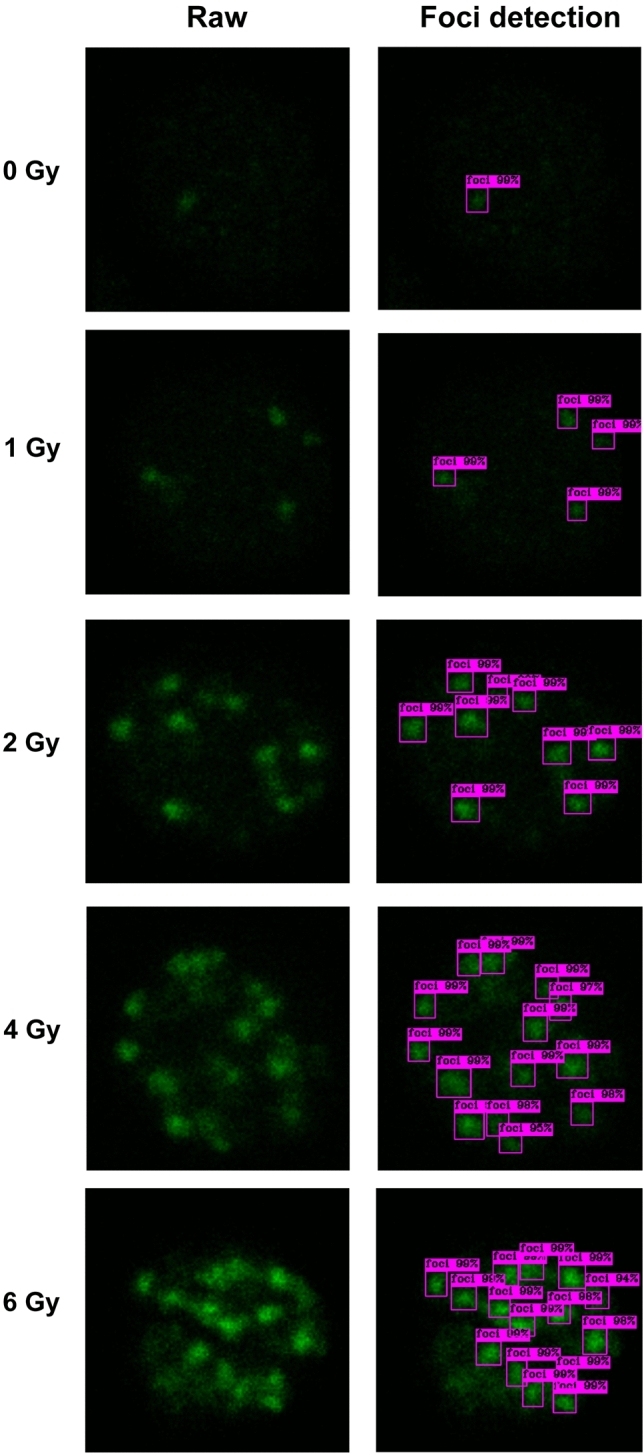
Performance testing of the FociRad. Green channel cropped images of cells irradiated with X-rays (0, 1, 2, 4 or 6 Gy) (left column) and automated foci detection with confidence thresholds by the FociRad model (right column) are shown.
